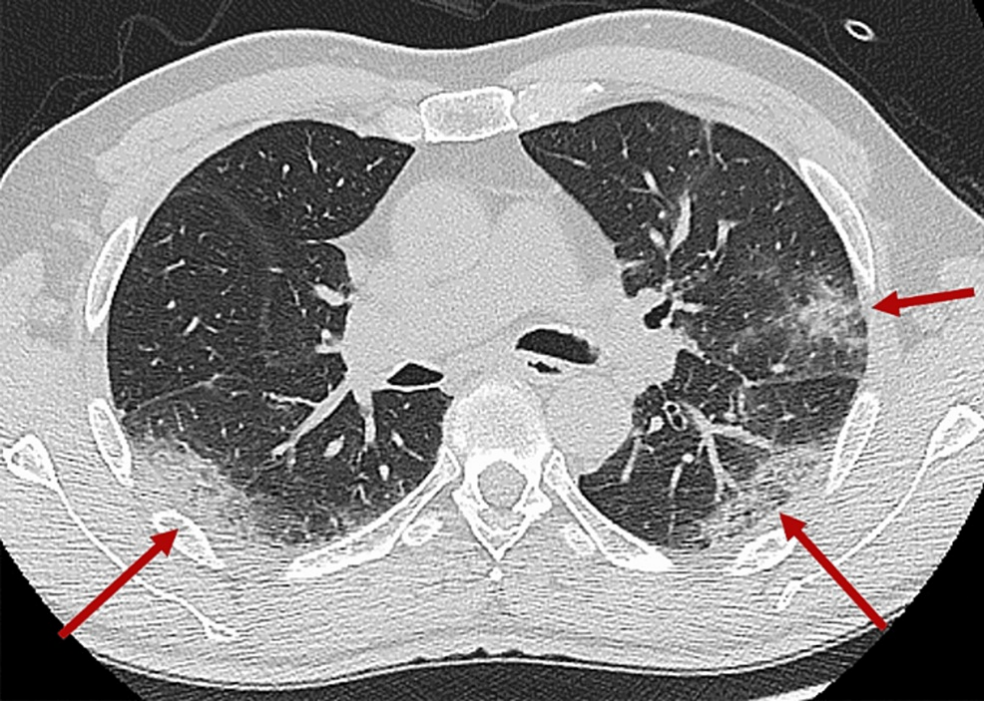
CT pulmonary angiography revealing a few bilateral interstitial infiltrates shown with red arrows.
